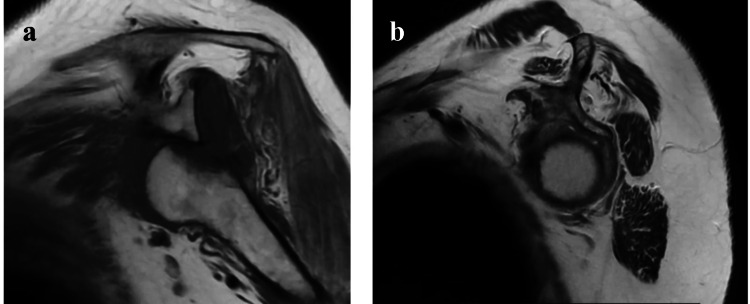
Left shoulder coronal magnetic resonance imaging (MRI) image. T2-weighted gradient echo axial MRI image showing accumulation of fat, 50% fatty muscle atrophy at the supraspinatus and infraspinatus which consists in a grade 3 of Goutallier classification and incongruent glenohumeral joint.
